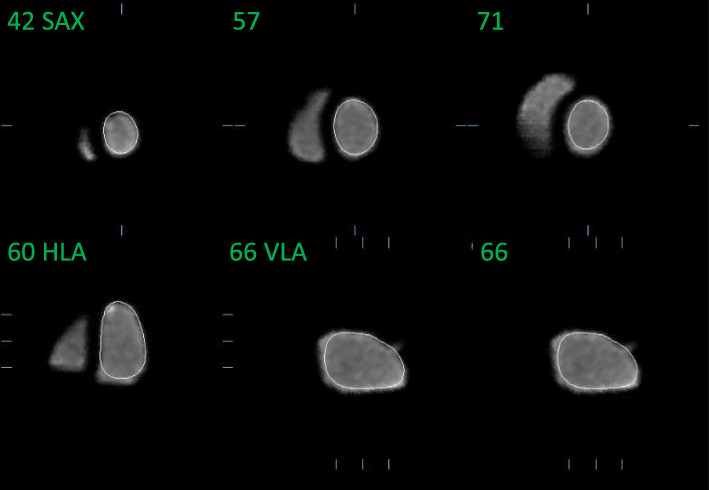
Automated segmentation with quantitative blood pool SPECT (QBS) of native 3D PET/CT images at end-diastole. The lines indicate the imprecise ventricle edges that were determined by this automated segmentation algorithm
